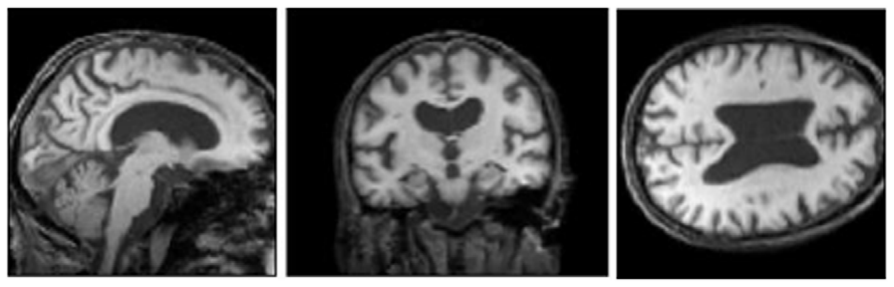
T1-weighted structural MRI of patient WH. MRI shows altered and increased ventricles on both sides. Informed consent was obtained for publication of this figure.
